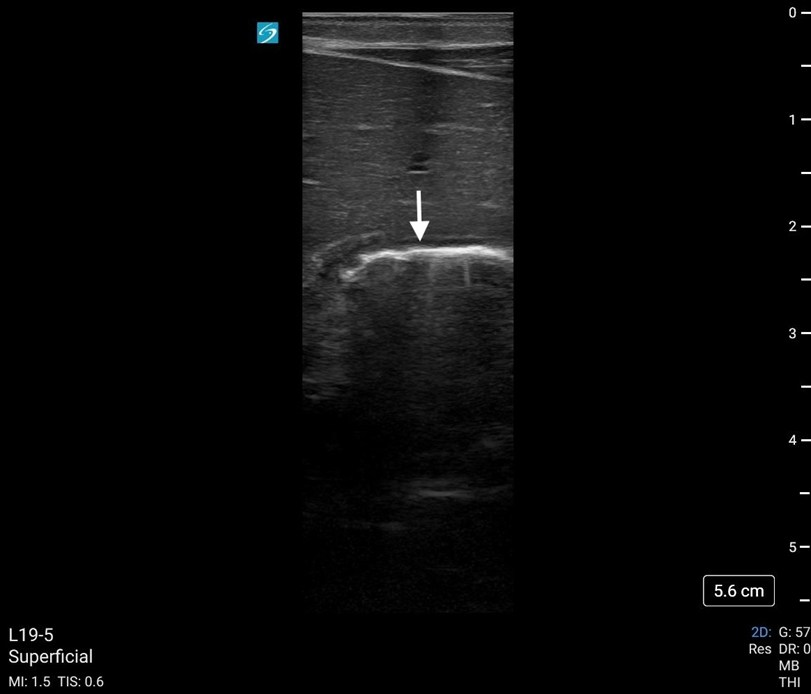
Gastric mucosa (white arrow) can be seen as a hyper-echoic structure. Reverberation artifacts and reported gastric and intestinal distention can lead to false-positive point of care ultrasound (POCUS) finding.
